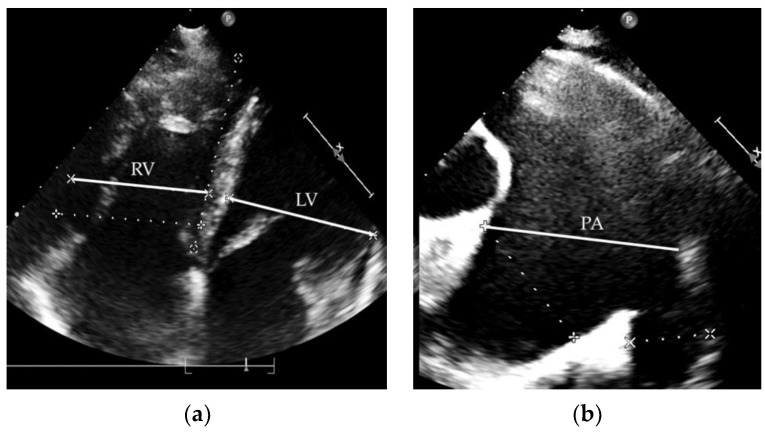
Patient 1 echocardiogram: (a) dilated right ventricle; (b) dilated pulmonary artery. RV—right ventricle; LV—left ventricle; PA—pulmonary artery.
